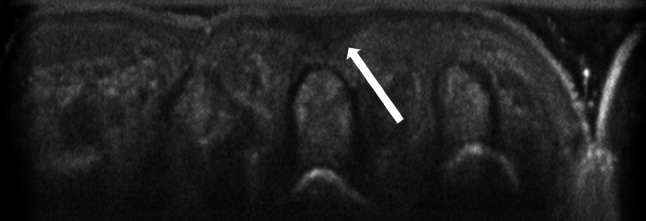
Representative picture of sonographic assessment for scar tissue. The *white arrow* indicates the triangular hypo-echoic scar tissue region
